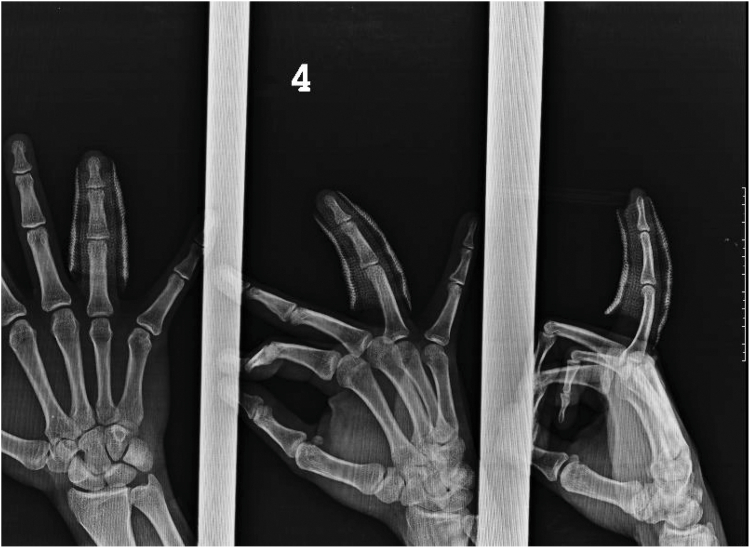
Two-week follow-up x-ray showing persistent fracture gaps
